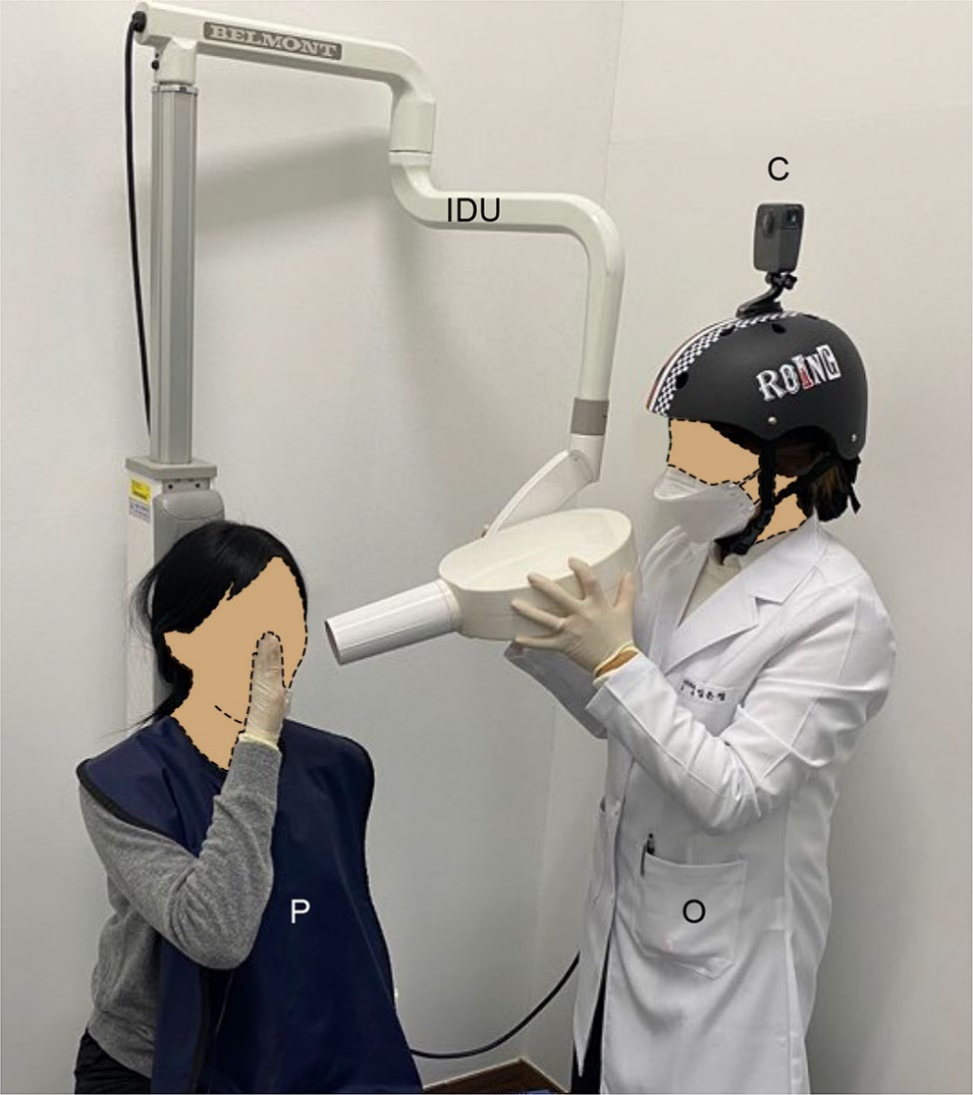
A 360° video recording. Dental radiography using the bisecting angle technique from the operator’s point of view using a 360° camera. The operator controls the intraoral dental X-ray unit to explain the dental radiography process. C: 360° camera, IDU: Intraoral dental X-ray unit, O: Operator, P: patient
